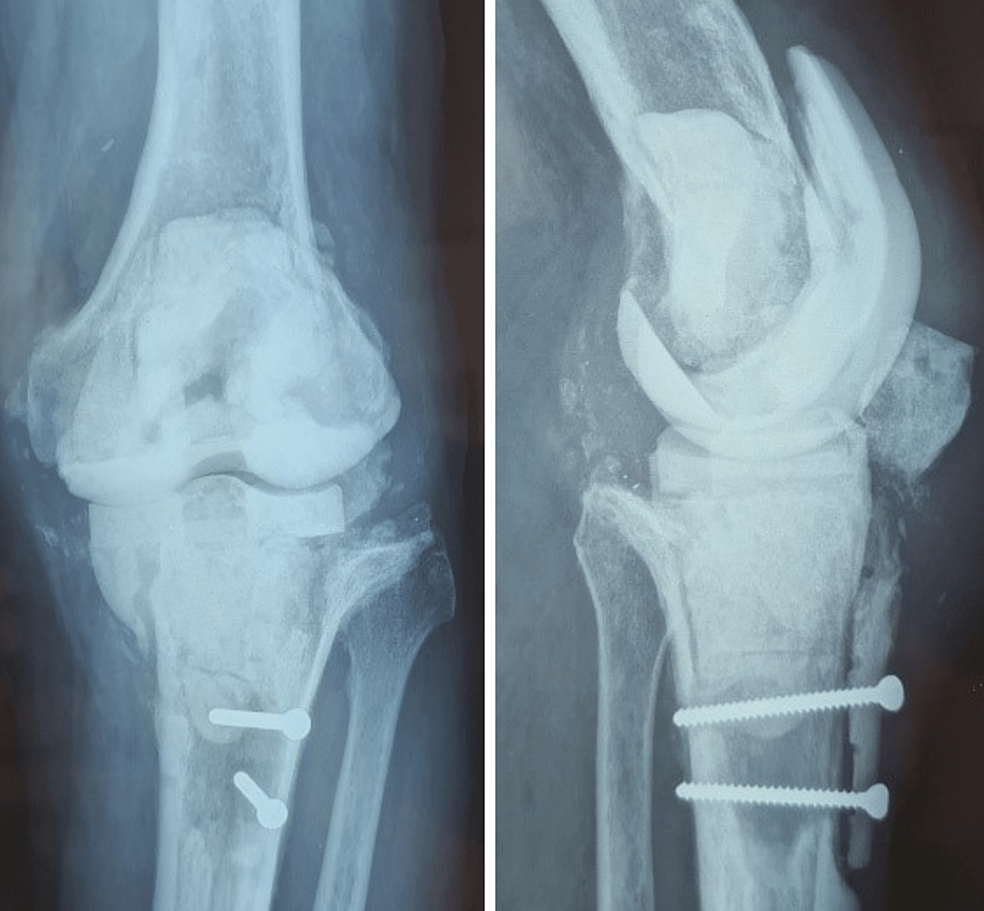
Antero-posterior (AP) and lateral (L) knee radiographs of the first stage revision of TKR
